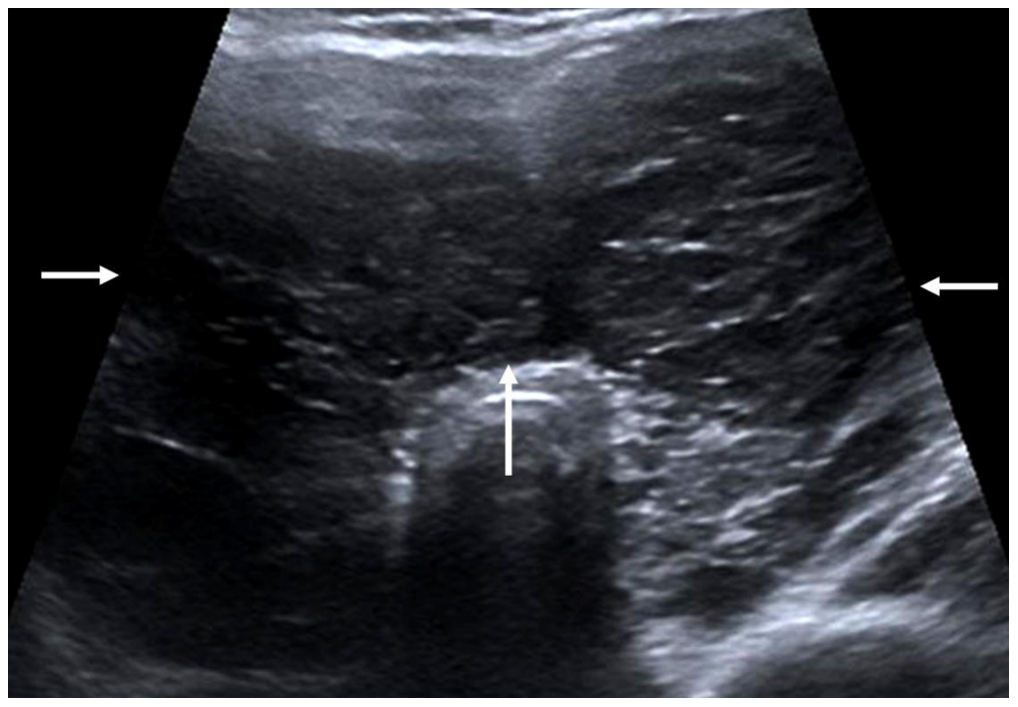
A 50-year-old female patient with diffuse PTL. Transverse sonogram shows the enlarged thyroid with decreased heterogeneous internal echoes (arrows).
